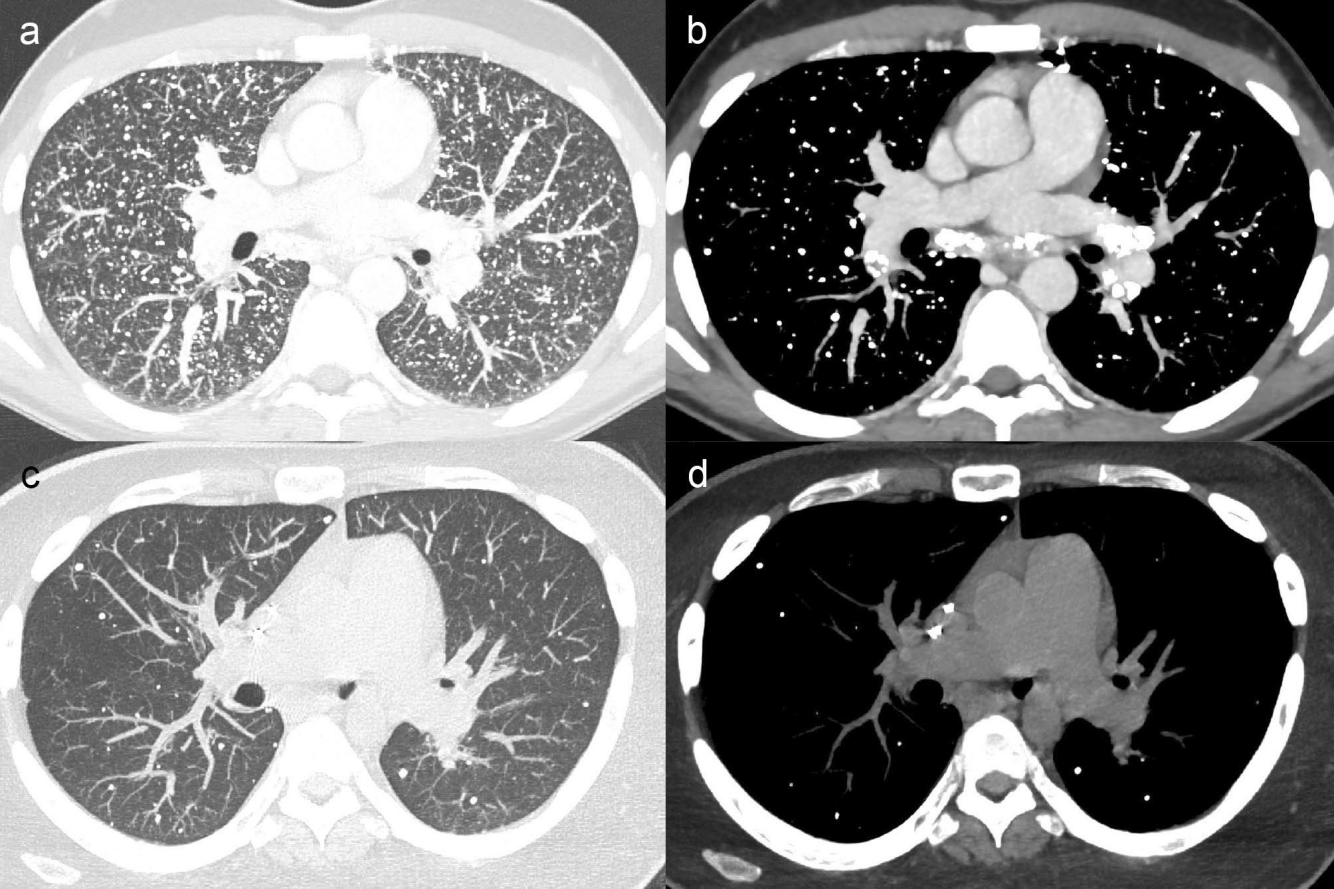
Calcified miliary metastases versus calcified nodules resulting from a previous infection. a, b Calcified metastases in a 33 y.o. man, who had undergone thyroidectomy due to a medullary thyroid cancer 10 months before. a Axial computed tomography (CT) maximum intensity projection (MIP) image depicts multiple sharply defined calcified micronodules with miliary distribution; note calcified hilar and mediastinal lymph nodes in b. Differential diagnosis with pneumoconiosis might be hard even though miliary distribution is less likely to be related to exposure, and patient’s age and history should always be taken into consideration. c, d Calcified nodules in a 38 y.o. man with previous varicella pneumonia. Axial CT MIP images show multiple calcified nodules and micronodules randomly distributed throughout the lungs, which are commonly found years after varicella infection
